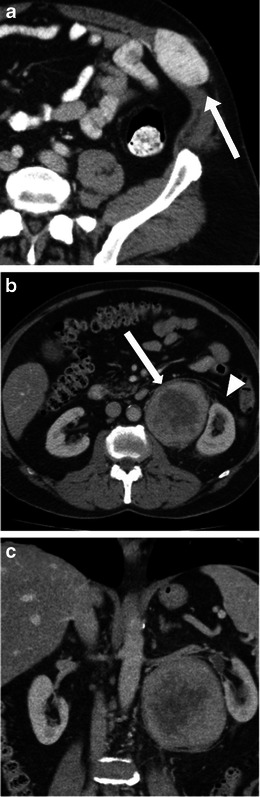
Imaging of solitary fibrous tumour (SFT). a A 40-year-old woman with a palpable abdominal wall. CT showed an intensely enhancing mass (*arrow*). SFTs in certain locations, such as the abdominal wall and the liver, are predominantly seen in female patients. b, c A 63-year-old man with a long-standing history of back pain. MRI of the spine incidentally picked up a mass in the retroperitoneum and CT of the abdomen was performed. Axial (b) contrast-enhanced CT shows a left sided large heterogeneous retroperitoneal mass (*arrow*) showing enhancement with central necrotic area, which is displacing and compressing the adjacent kidney causing mild hydronephrosis (*arrowhead*). Coronal CT (c) in the same patient shows the large mass causing distortion of the left kidney and left renal vessels
